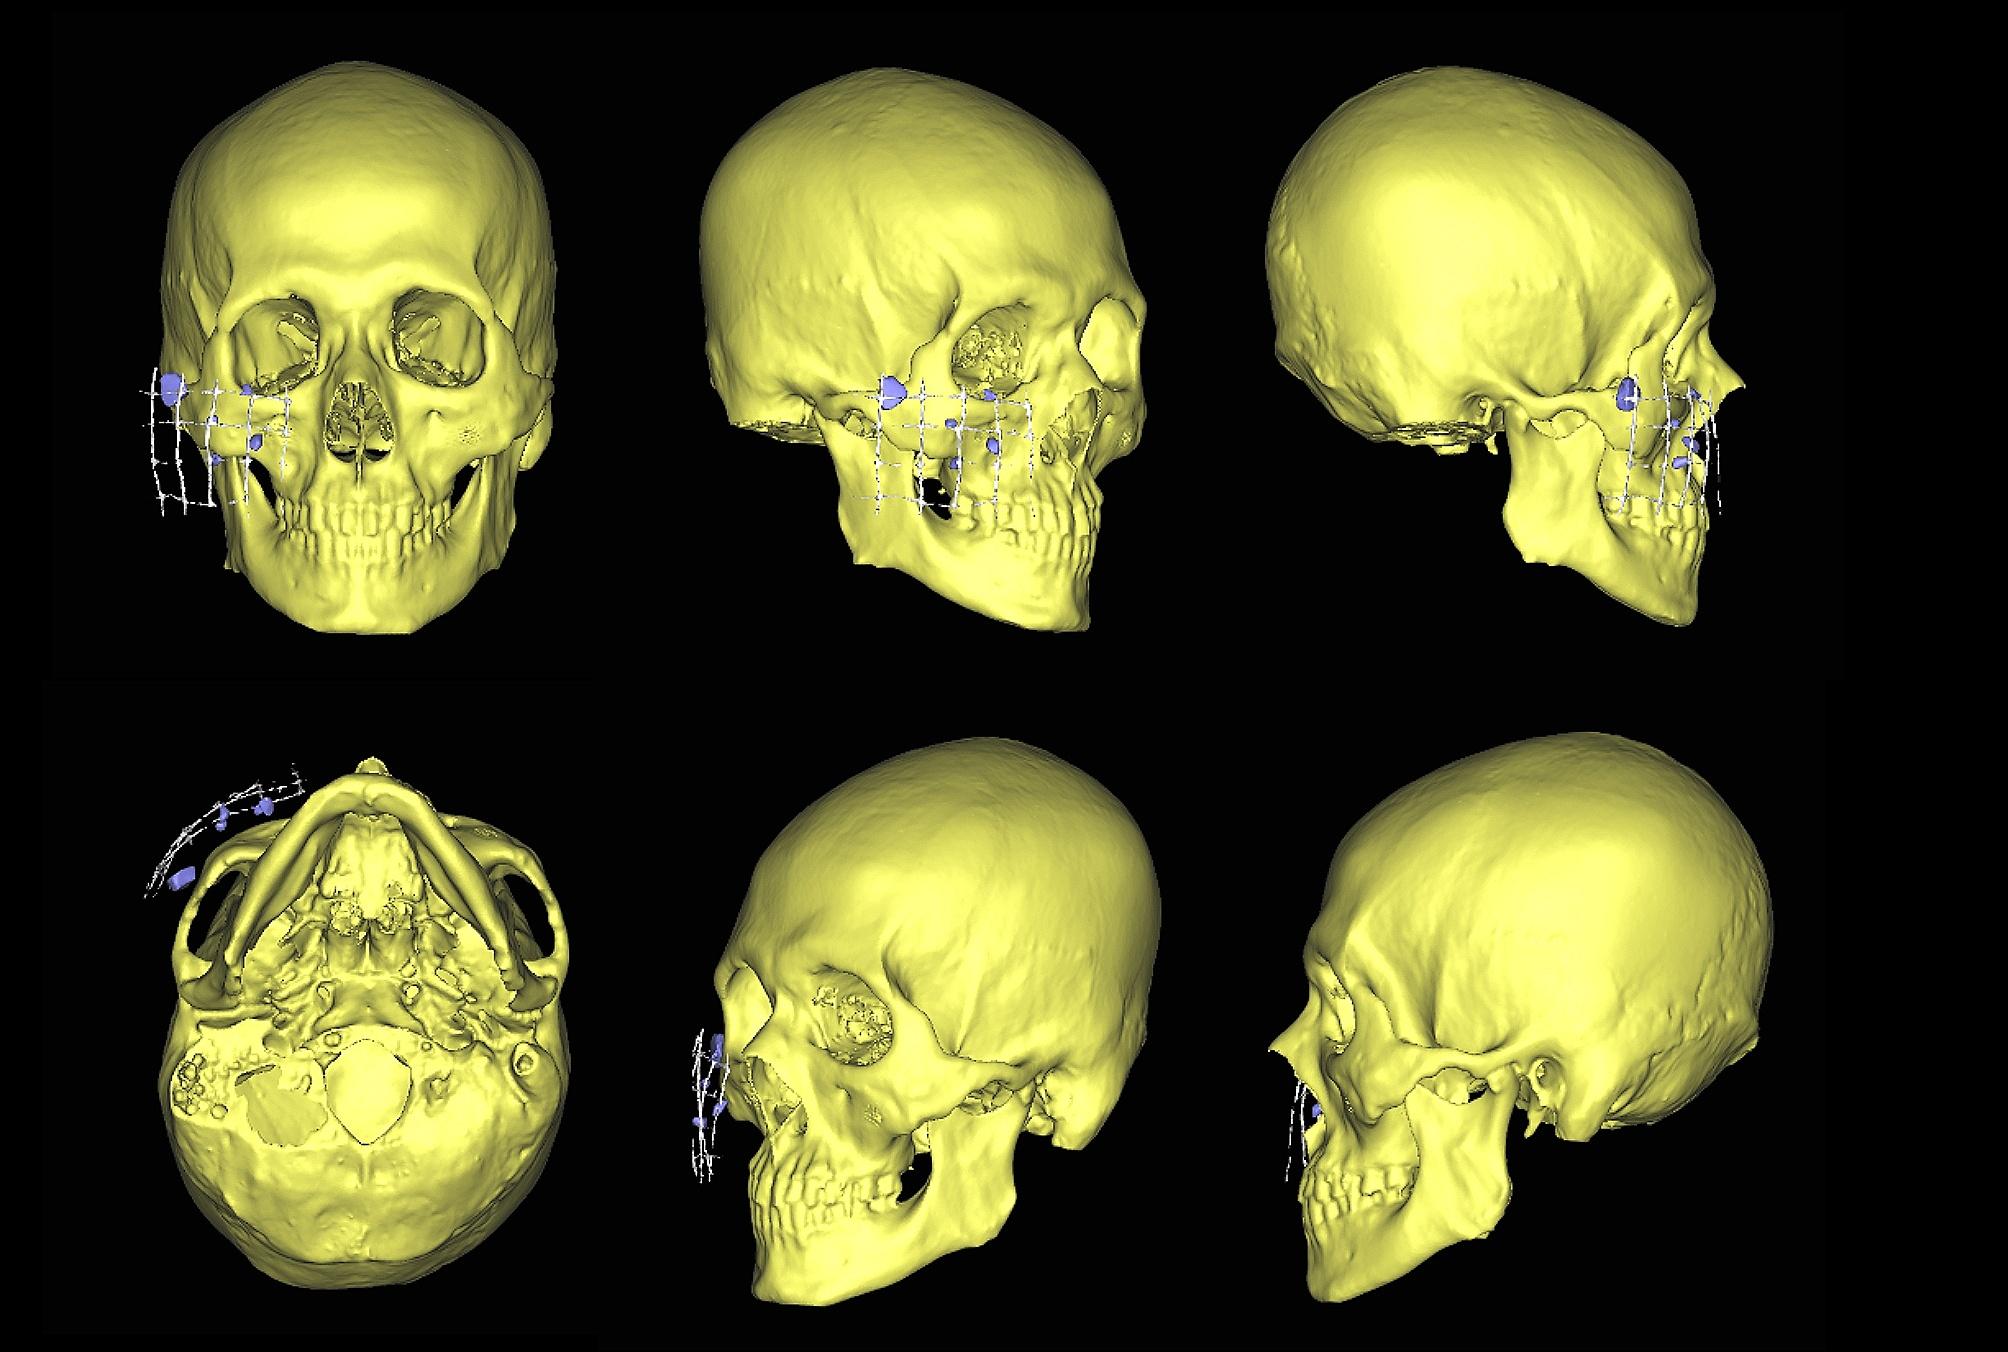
After CT scan, DICOM data was imported into Proplan software to perform the analysis. Segment FBs, reference grids and bone and create 3D model
